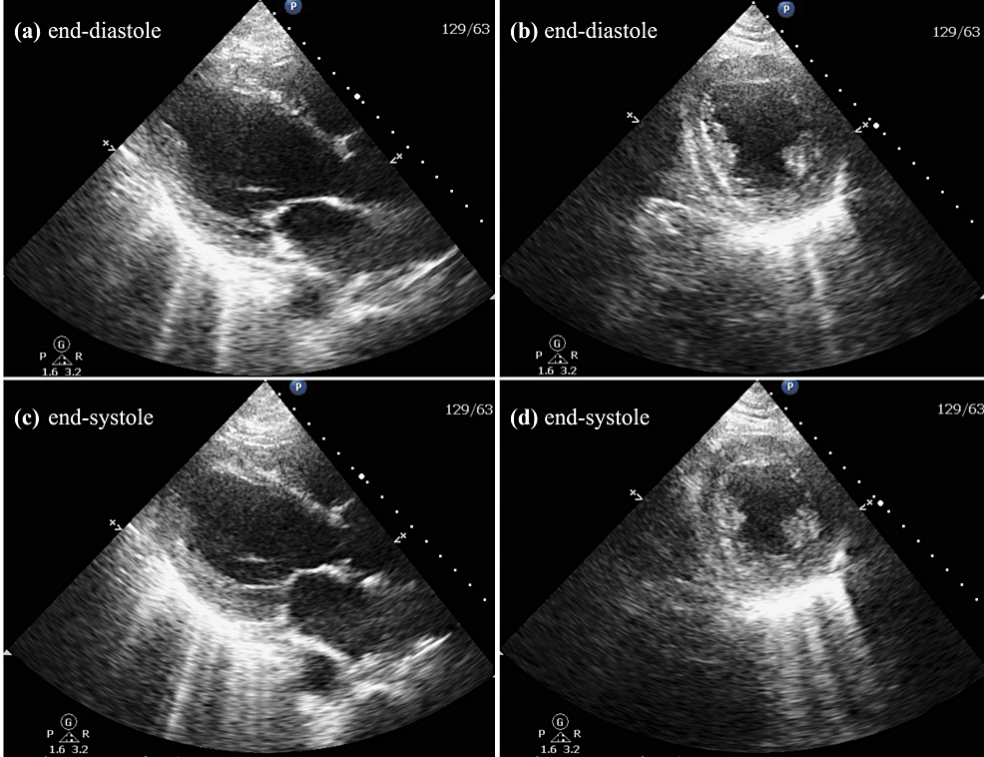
Transthoracic echocardiogram two months after the initiation of bicalutamide Transthoracic echocardiogram parasternal long axis and short axis views two months after the initiation of bicalutamide. (a) and (b): end-diastole long axis and short axis views, respectively; (c) and (d): end-systole long axis and short axis views, respectively. The left ventricular ejection fraction was 15% with severely decreased systolic function and mildly dilated left ventricle but normal left ventricular wall thickness
